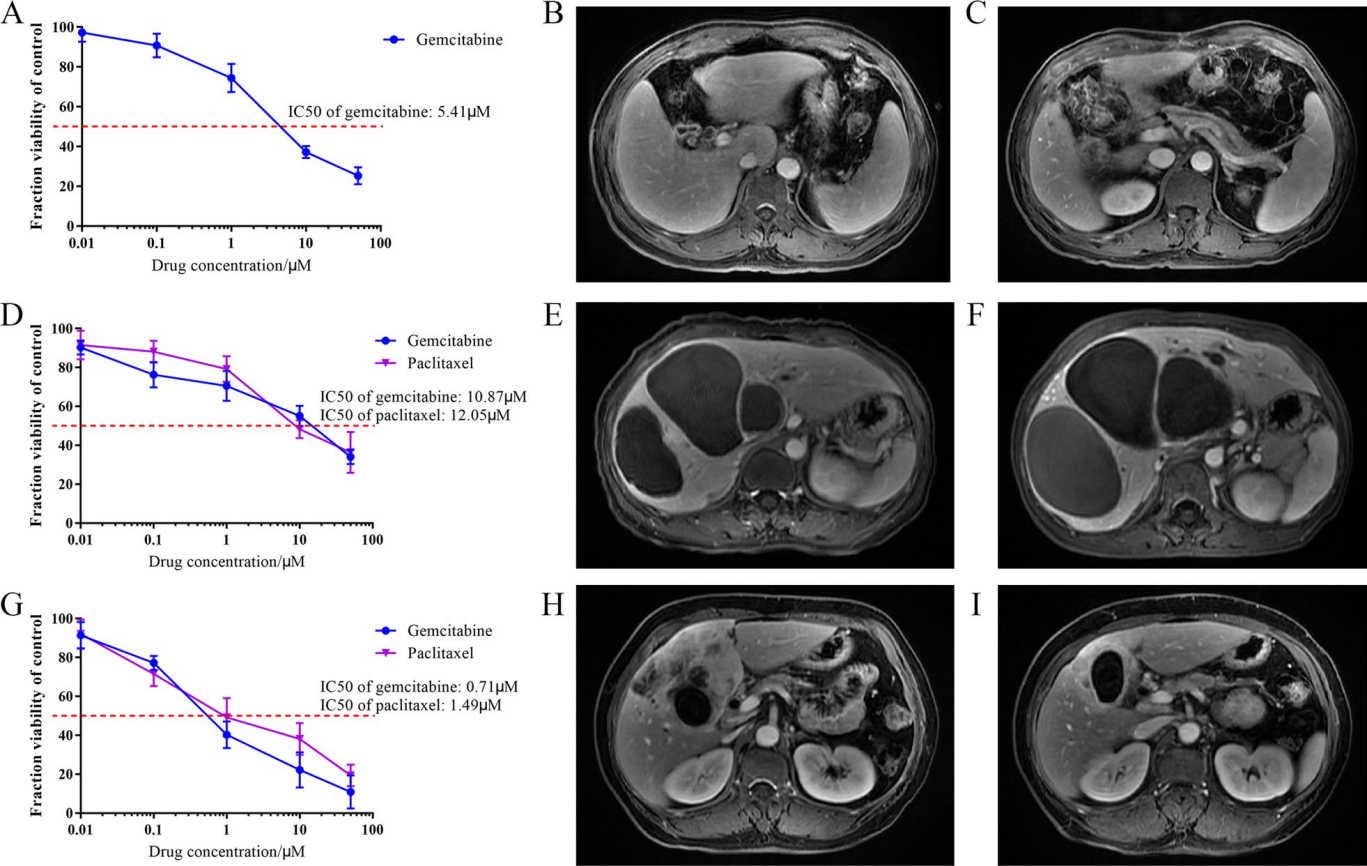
Three patients receiving perioperative adjuvant treatment. A Dose-response curve of gemcitabine in eCCA PDO is presented. B, C The eCCA patient was diagnosed as eCCA on October 8, 2020 according to MR images (B) and received surgical resection (C). D Dose-response curve of gemcitabine and paclitaxel in GBC1 PDO is presented. E, F The GBC1 patient was diagnosed as GBC on June 11, 2020 according to MR images (E). Then gemcitabine and albumin-bound paclitaxel were given for two cycles but MR images indicated the tumor had progressed according to MR images (F). G Dose-response curve of gemcitabine and paclitaxel in GBC2 PDO is presented. H, I The GBC2 patient was diagnosed as GBC on August 7, 2020 according to MR images (H) and received neoadjuvant chemotherapy of gemcitabine plus albumin-bound paclitaxel plus tislelizumab for 12 cycles, showing partial response (I)
